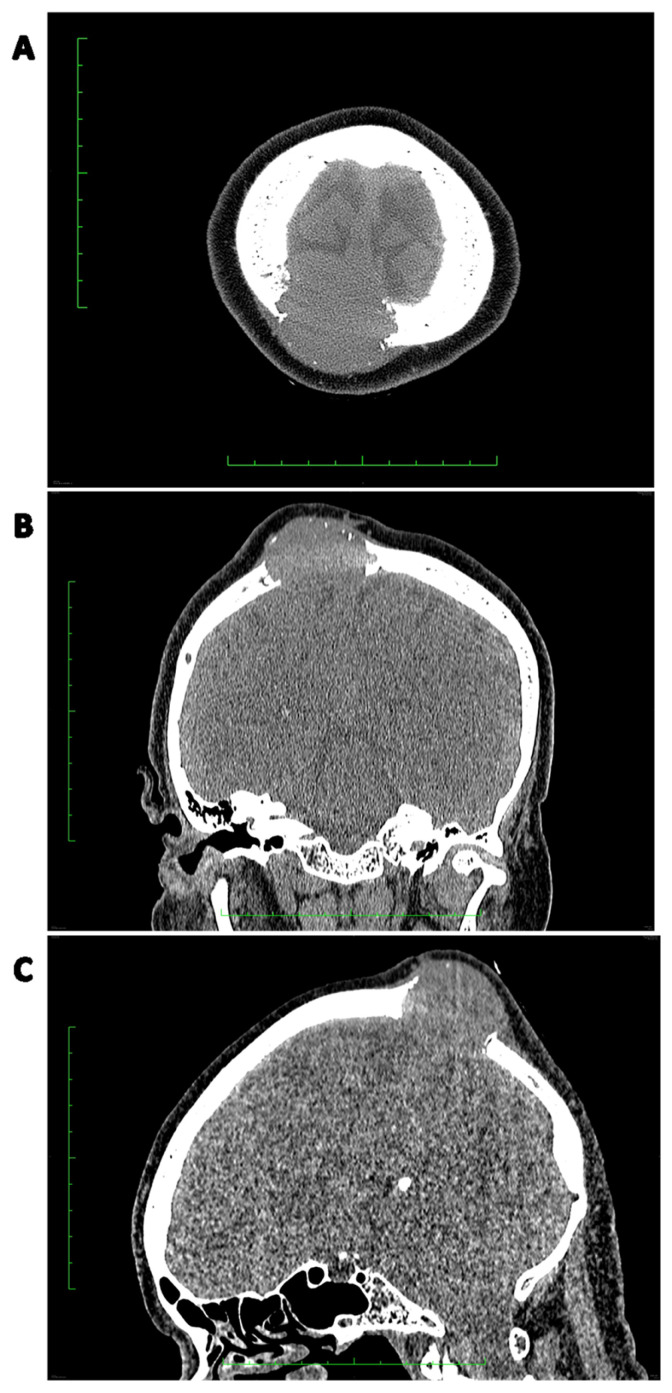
A 65-year-old patient with locally advanced SFT in parietal bone with infiltration of the superior sagittal sinus with adhesion to the dura mater was admitted in 2022 to begin systemic treatment. Symptoms had begun in March 2019, with a growing subcutaneous tumor in the parietal area. It was initially excised by a dermatologist and diagnosed as lipoma. In February 2022, due to tumor recurrence, the patient underwent a biopsy which was diagnosed as a malignant solitary fibrous bone (SFTB). (A) CT scan, axial view showing the destruction of the parietal bone. (B) CT scan, coronal view showing bone destruction with a soft tissue mass adhering to the skin. (C) CT scan, sagittal view clearly depicting the size of the tumor in relation to the parietal bone and skin.
